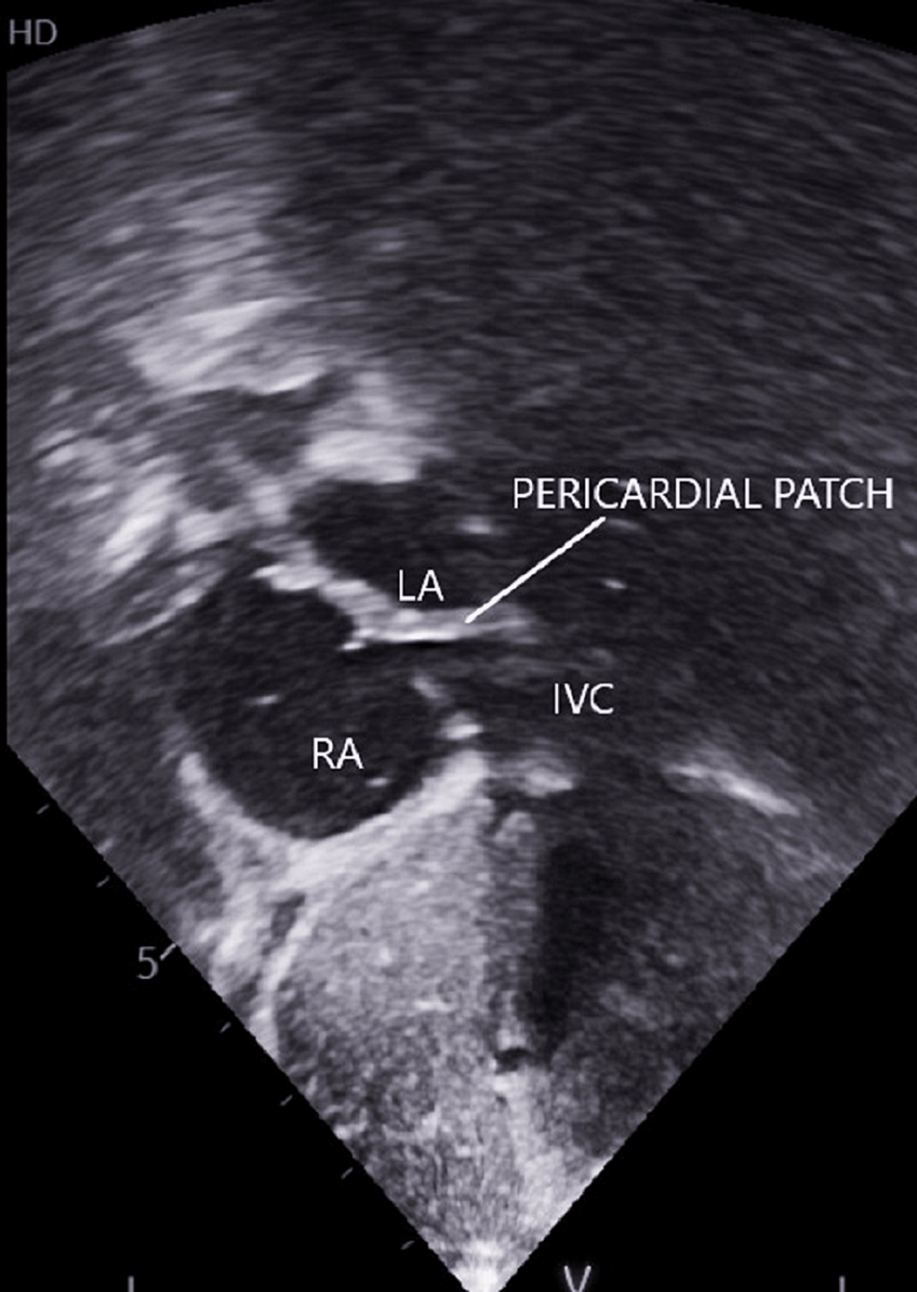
Case 1 - Postoperative echocardiography showing the oblique orientation of the pericardial patch. LA: left atrium; RA: right atrium, IVC: inferior vena cava
